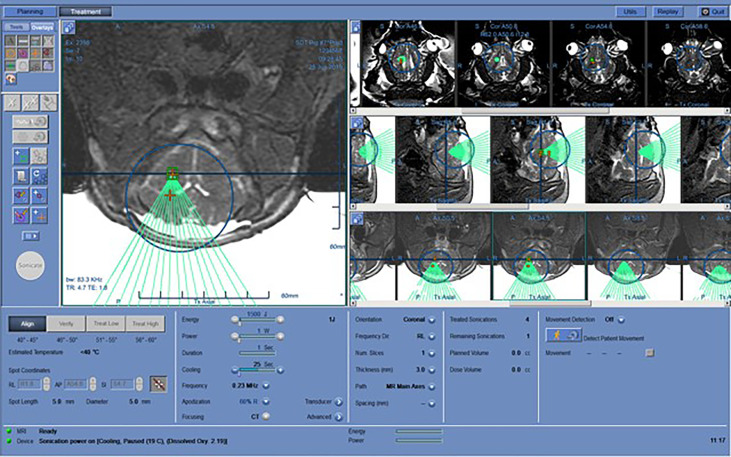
Working layout of the Exablate system during insonation of one subject. The insonation pattern is superimposed on the pre-acquired MR images in different slices.
